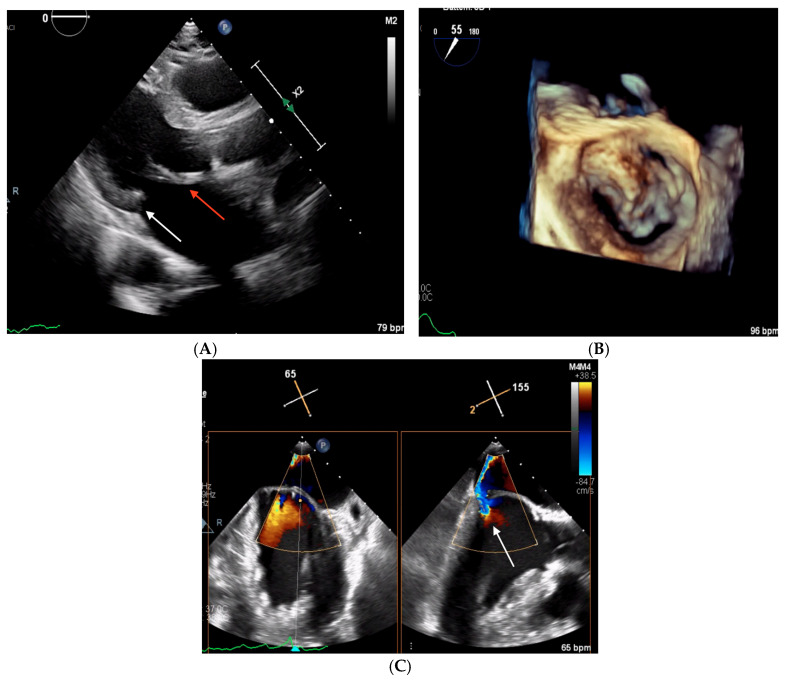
Echocardiographic images obtained in Clinical Case 3. (A) TTE parasternal long-axis view showing a thickened and elongated AML (red arrow) and the hypoplastic PML (white arrow). (B) 3D TEE “en face” view of mitral valve showing a severely hypoplastic PML and an AML prolapsing in A1 and A3. (C) Color Doppler 2D TEE 65° mid-esophageal view (left panel) and the orthogonal plane crossing A2/P2, where the origin of the MR jet is located (right panel, white arrow).
